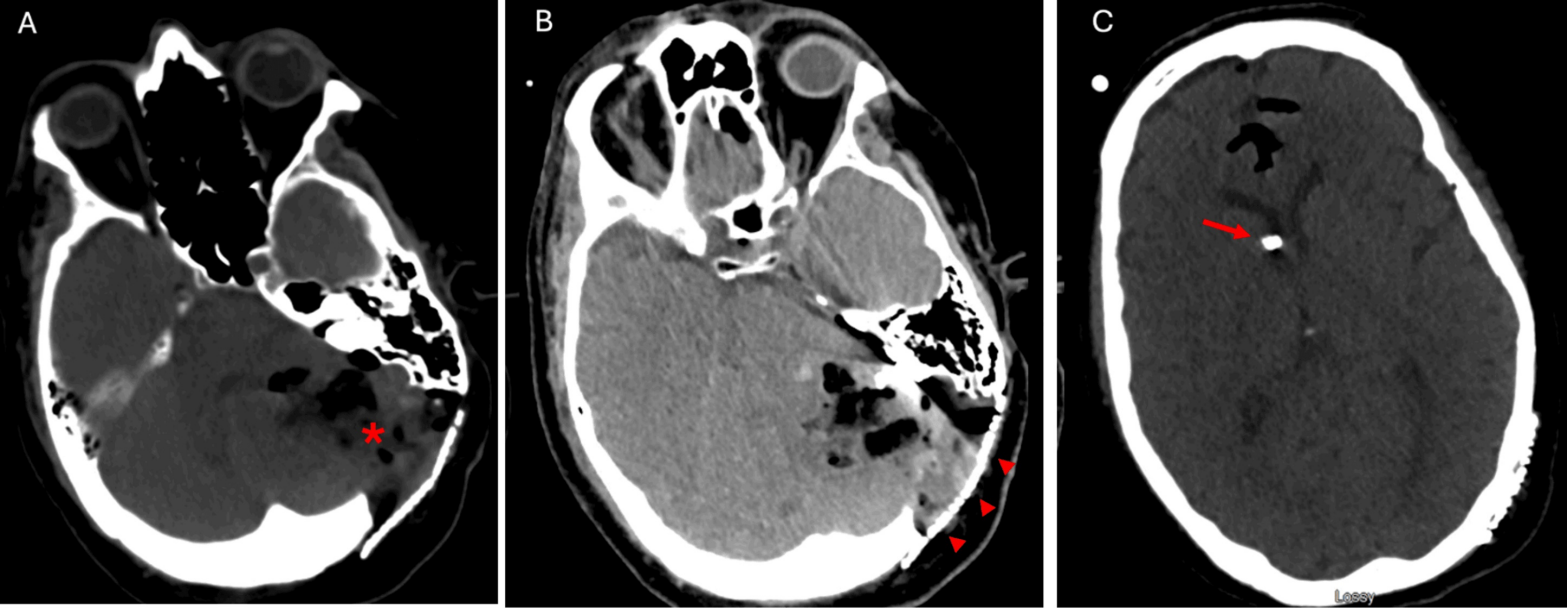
The patient's postoperative computed tomography of the head (CTH) Non-contrast axial CTH demonstrating interval removal of left cerebellar mass lesion with surgical bed predominantly occupied by multiple gas pockets (panel A, red asterisk) post suboccipital craniectomy with mesh cranioplasty (panel B, red arrowhead). Placement of right frontal external ventricular drain, with the tip terminating in the lateral ventricle near the right foramen of Monro (panel C, red arrow).
